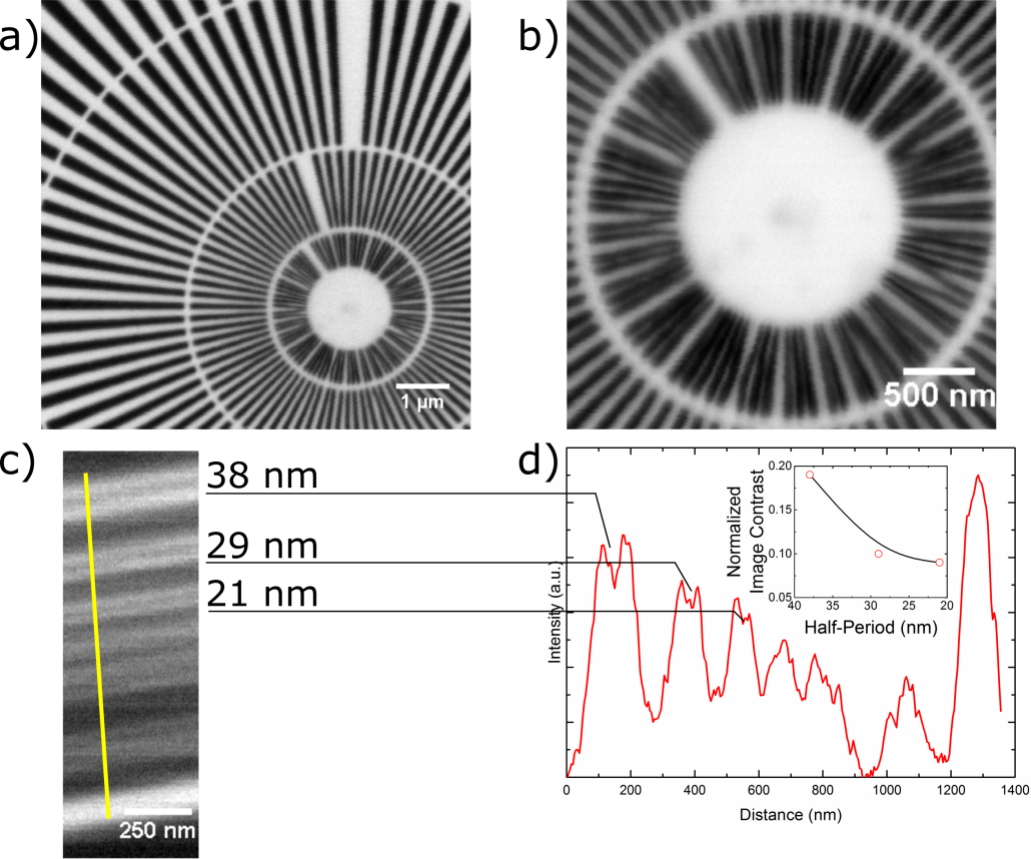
a) Soft X-ray image of the Siemens star recorded at 1 keV with 0.94 ms pixel dwell time and 11 nm step size. b) A higher-magnification image of the central ring recorded using 10 nm steps size and 10 nm dwell time. It can be seen that the smallest 30 nm features of the Siemens star are clearly resolved. c) An STXM image of the multilayer test object recorded at 1.12 keV with 30 ms dwell time and 5 nm step size. The 21 nm half-pitch features are resolved and the intensity profile in d), where the inset shows the normalized contrast of the first three features in the profile.
